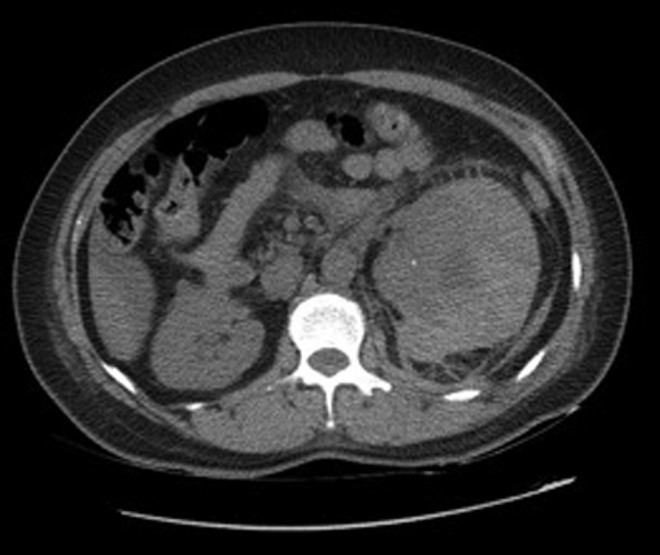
CT scan showing subcapsular hematoma with compression of the kidney.
